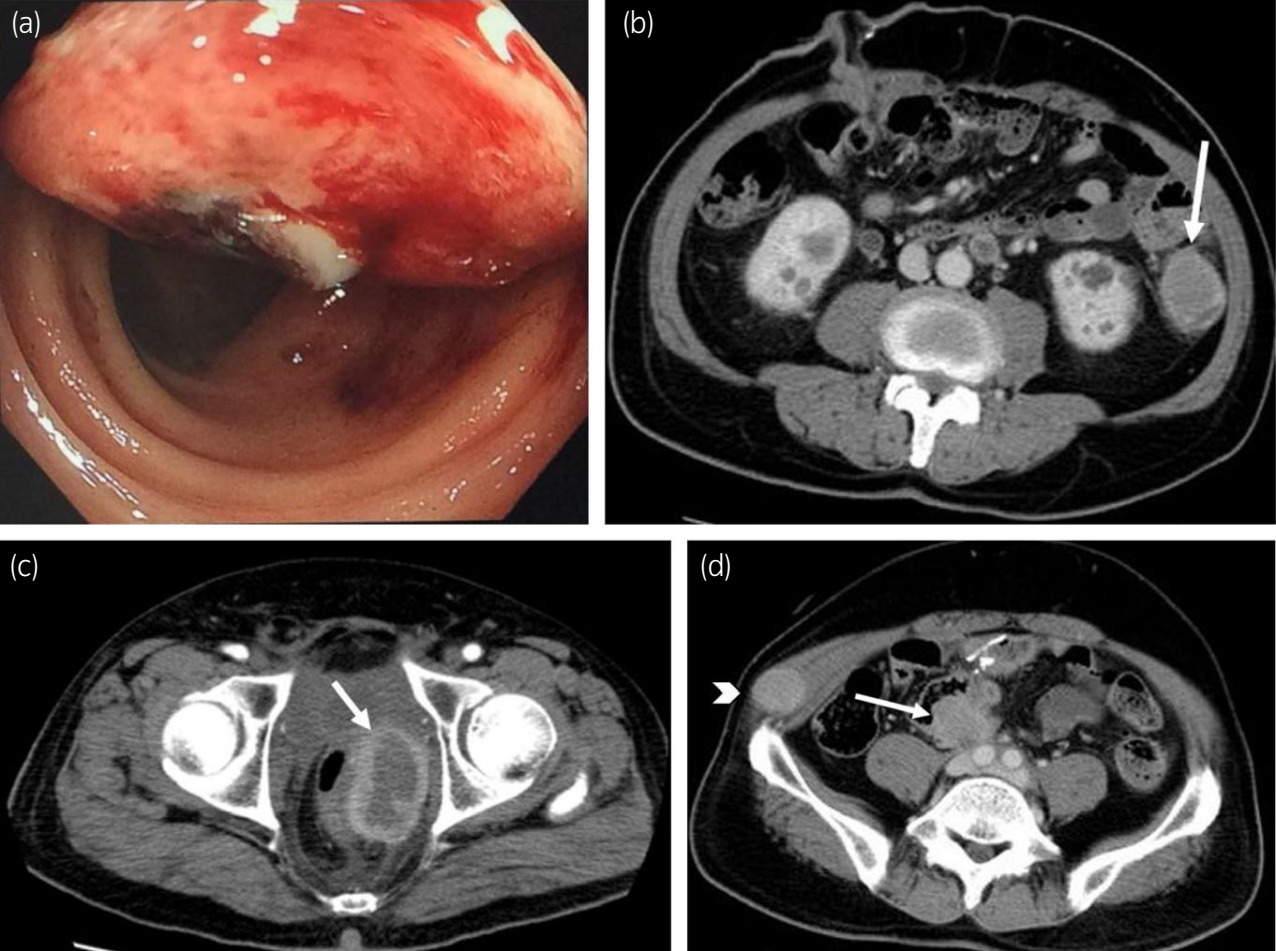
Multiple recurrences of bladder cancer after RARC; (a) colonoscopic findings showing an oozing submucosal tumor in the descending colon, (b–d) CT scan findings: (b) a 4‐cm intraperitoneal tumor in the descending colon (arrow), (c) a huge local recurrent mass in the pelvic floor (arrow) (d) para‐aortic lymph nodes (arrow) and nodules in the oblique abdominal muscles (arrowhead).
